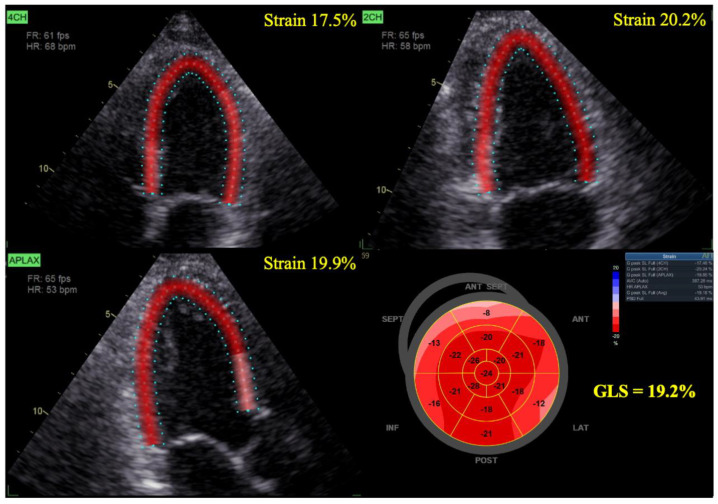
Measurement of GLS. Measurement of GLS by speckle−tracking echocardiography, where the regional strain map is superimposed on grayscale two−dimensional echocardiographic images in three apical long−axis views. GLS, global longitudinal strain.
